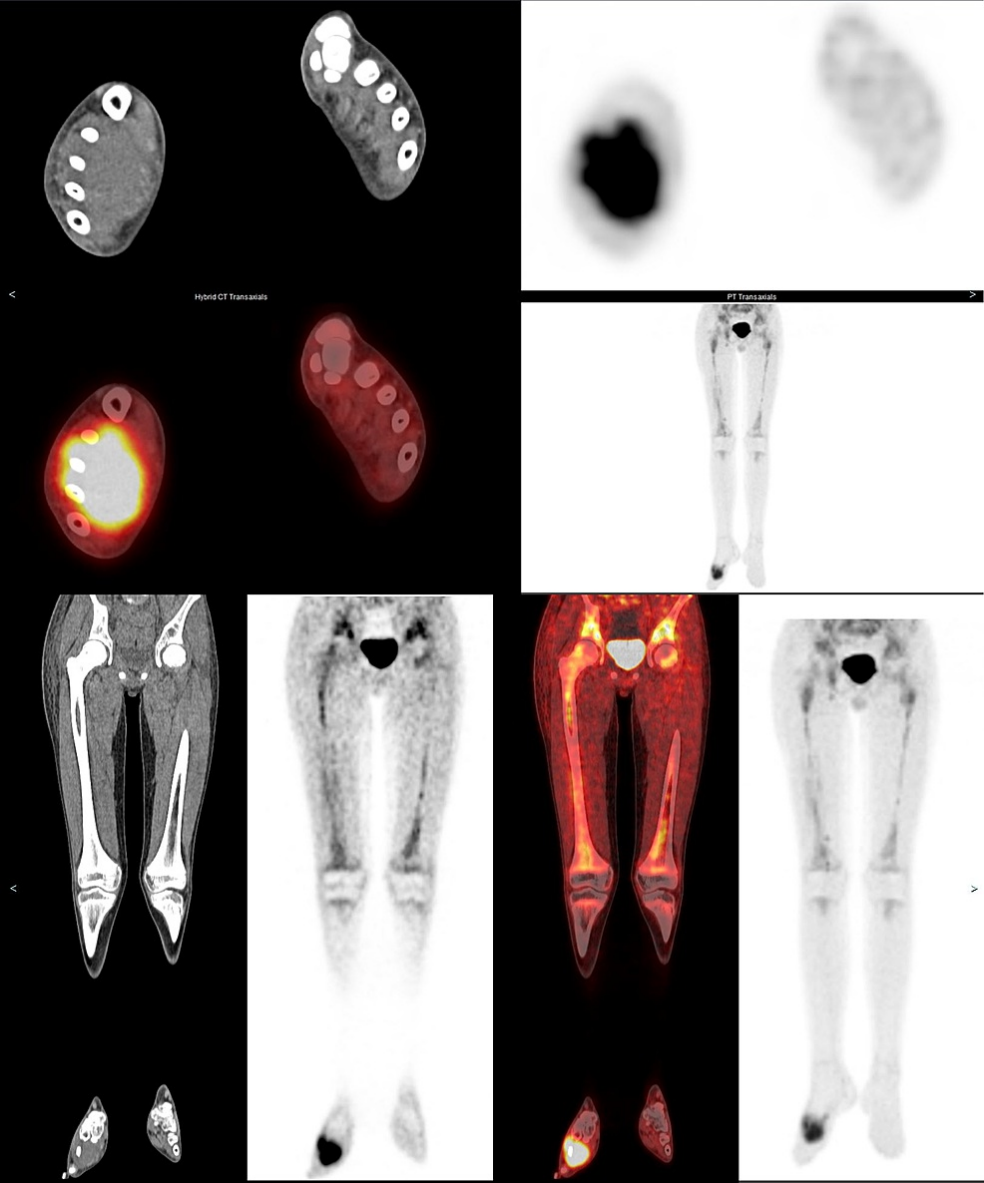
Lower leg axial and coronal PET-CT (non-enhanced CT, attenuation correction PET slice, and fusion images, from left to right) showed hypermetabolic soft tissue mass in the right foot. Coronal PET-CT images and maximum intensity projection (MIP) image (last image, from left to right) confirmed diffuse hypermetabolic marrow in femori bilaterally. Overall, this is concerning for primary mass in the foot with diffuse axial and appendicular marrow metastatic disease.
